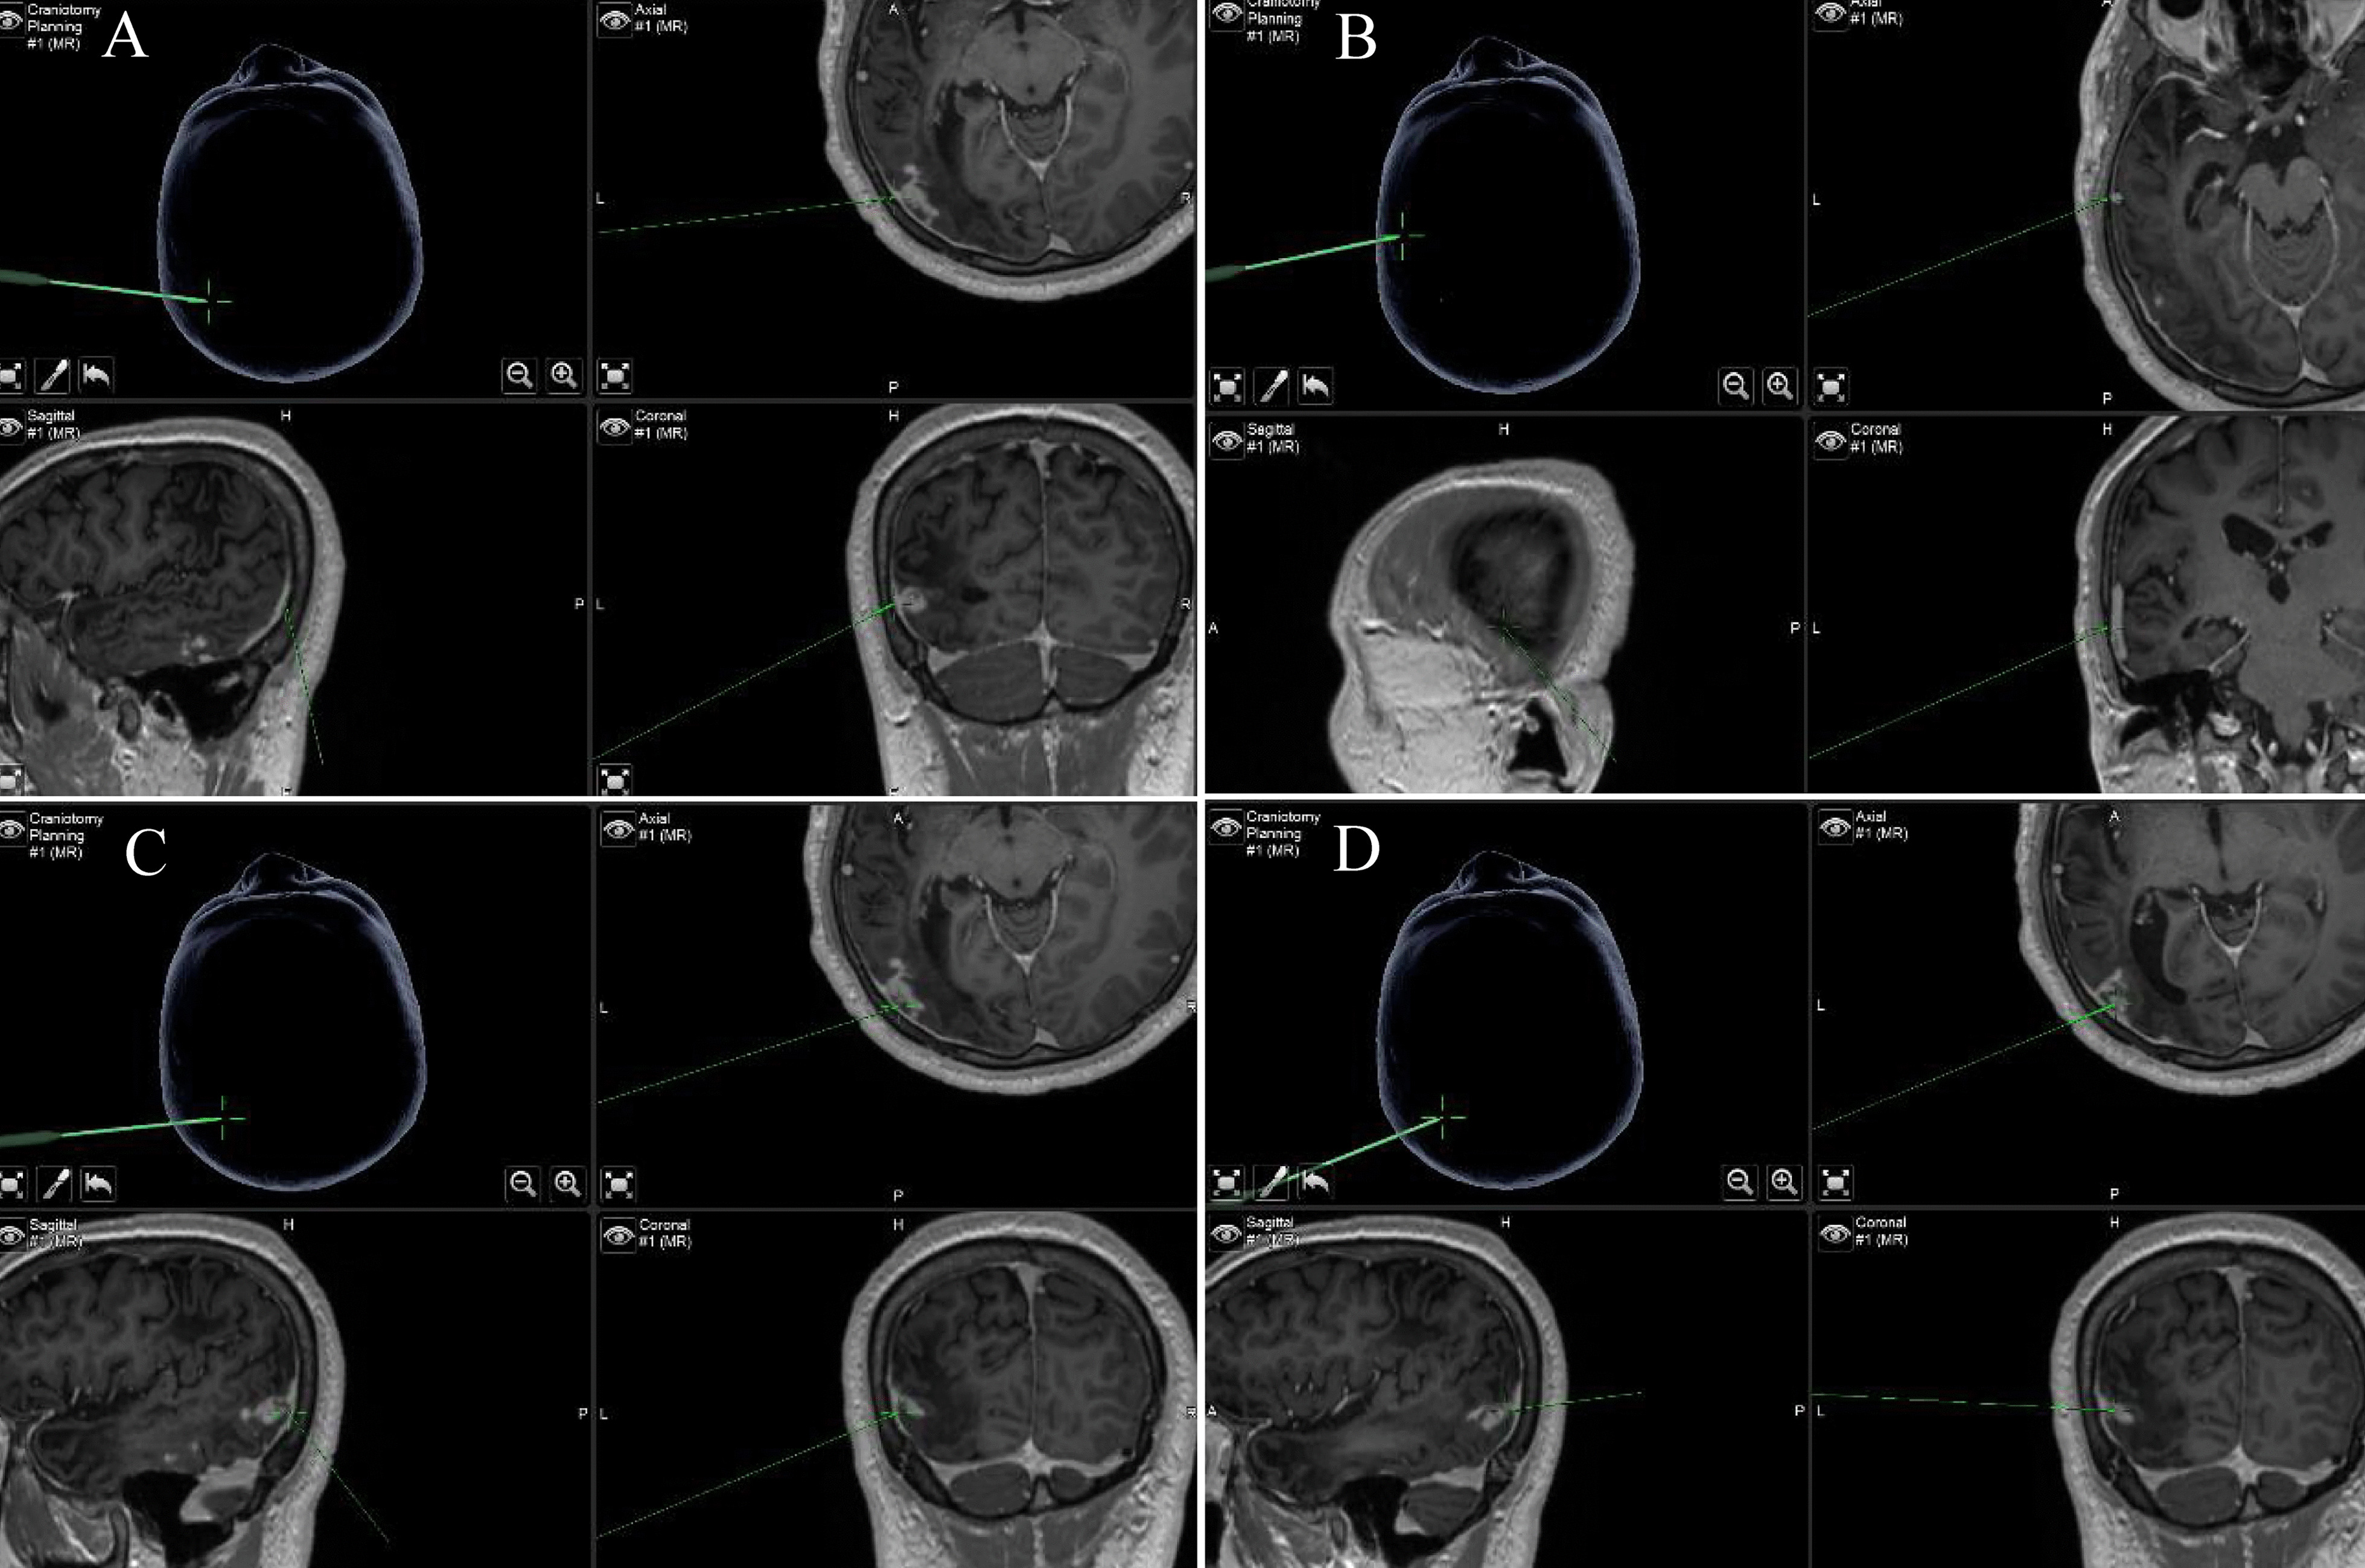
Neuronavigation and localization. (A–D) Neuronavigation combined with contrast-enhanced MRI to pinpoint lesions from sagittal, axial, and coronal, as indicated by green arrows
